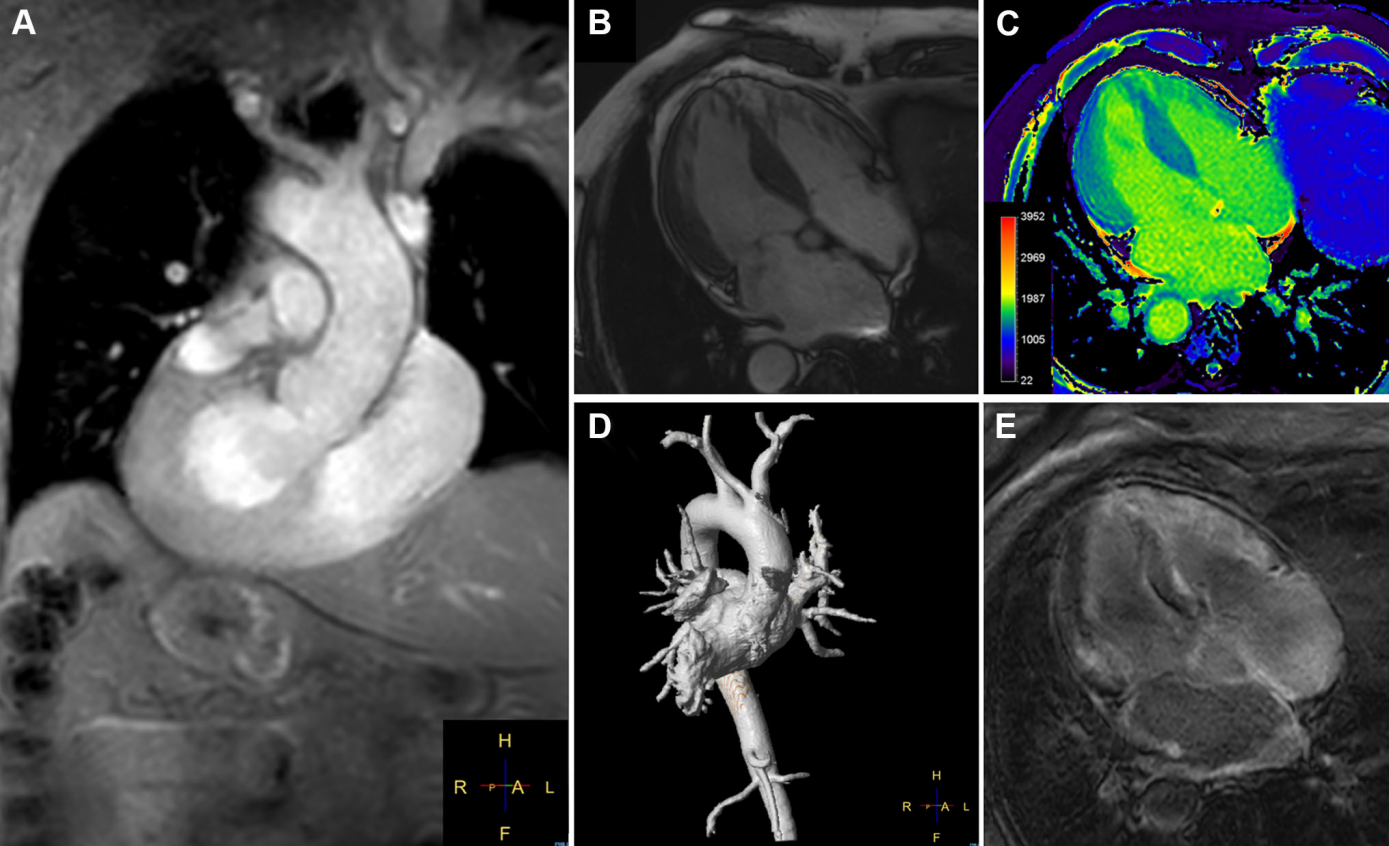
a Images from a cardiac MRI confirms a situs inversus with dextrocardia. The outflow tract of the left ventricle, the left atrium and the right-sided aortic arch are displayed. b Four-chamber view with the mirrored left and right ventricle and thickened left ventricular wall. c Contrast-free T1-mapping reveals elevated values for T1-times indicating increased amount of diffuse myocardial fibrosis. d Three-dimensional reconstruction shows the aorta and pulmonary trunk. e Late gadolinium enhancement of the left ventricle demonstrates high signal intensity patterns, typical for a clinical picture of cardiac amyloidosis
